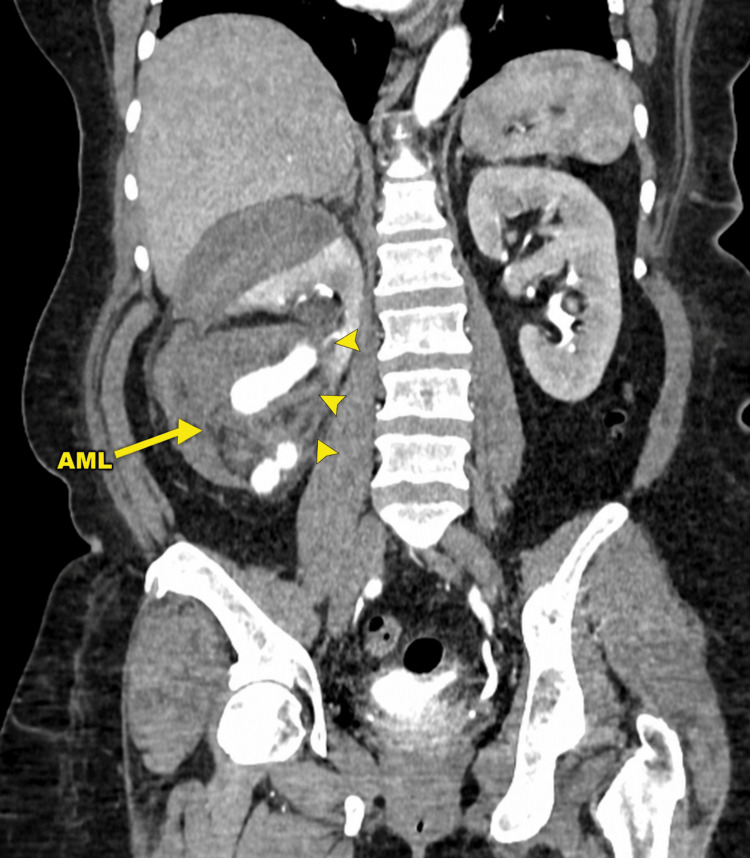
Coronal CT angiography images confirming the 12 x 14 cm right perirenal hematoma A heterogeneous, fat-containing mass consistent with an angiomyolipoma (arrow) is identified at the inferior pole of the right kidney, with associated giant aneurysms and pseudoaneurysms (arrowheads). AML: Angiomyolipoma
